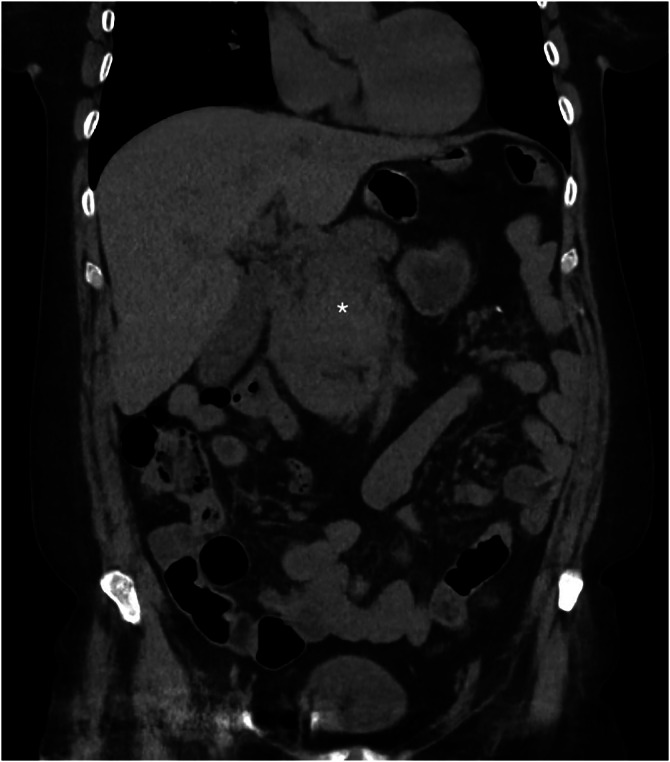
Non-contrast CT abdomen pelvis shows thickening of the pancreatic body (asterisk) with peripancreatic inflammatory changes.
